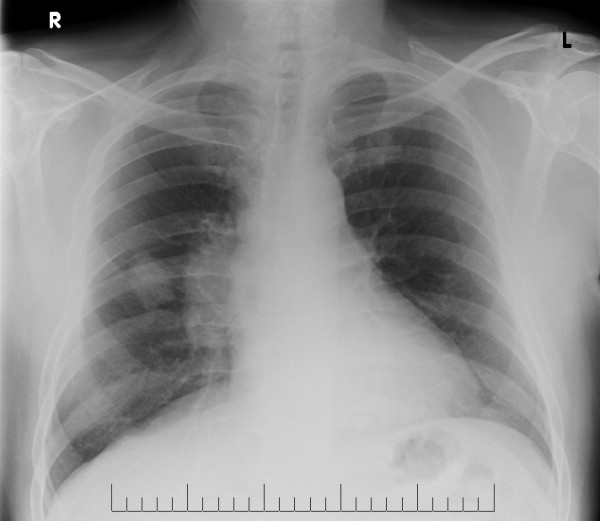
Chest X-ray showing the primary tumour in the right lung.
